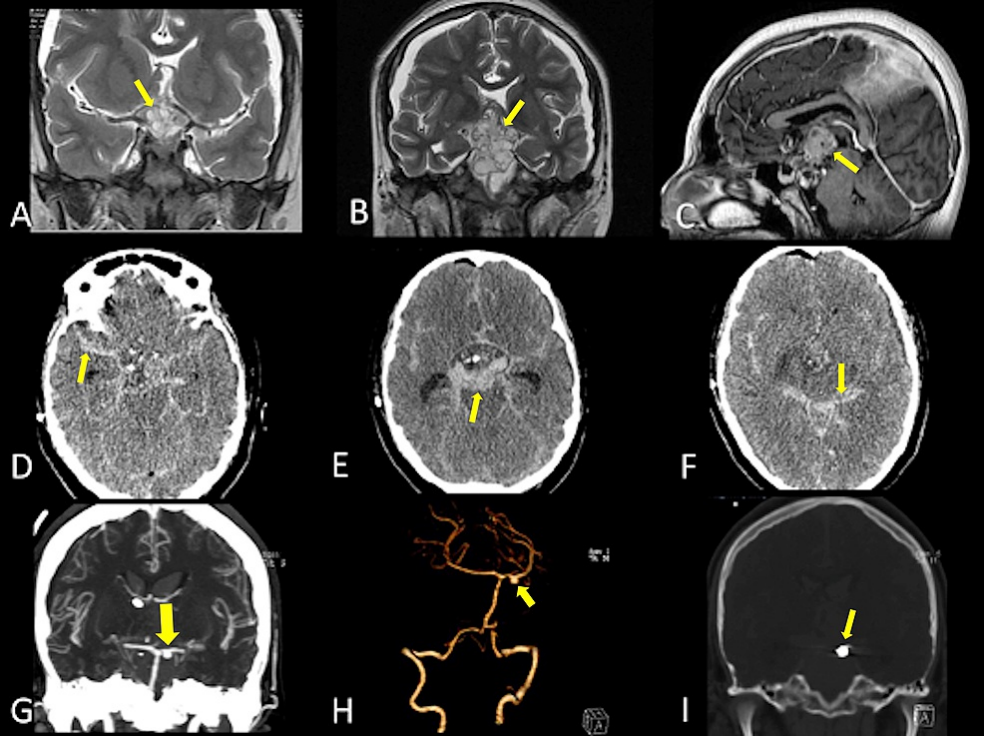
Case 2 representative MRI and CT angio images. (A-C) MRI shows a suprasellar lesion extending upwards. A retrosellar extension of the tumor is visible (arrows). (D-F) During surgery, severe bleeding was noticed and controlled. CT scan shows a generalized SAH with thick blood clots at the prepontine cistern (arrows). (G,H) CT angio shows a right small P1 aneurysm (arrows). (I) The aneurysm was treated by coiling (arrow). CT, computed tomography; MRI, magnetic resonance imaging; SAH, subarachnoid hemorrhage.
